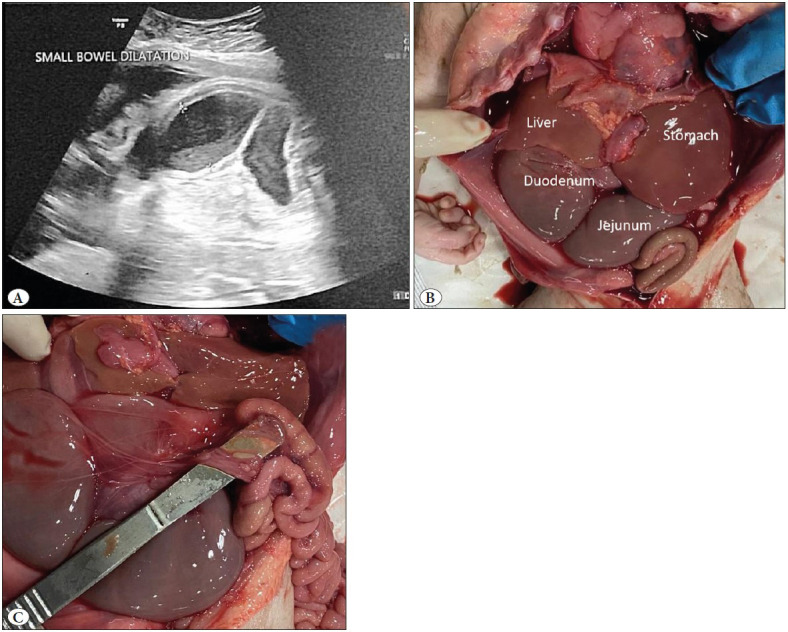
A) Ultrasound scan showing dilated gastric fundus bubble with dilated proximal bowel. B) Dilated and congested stomach, duodenum and jejunum. C) Scalpel head showing atretic intestinal segment.
